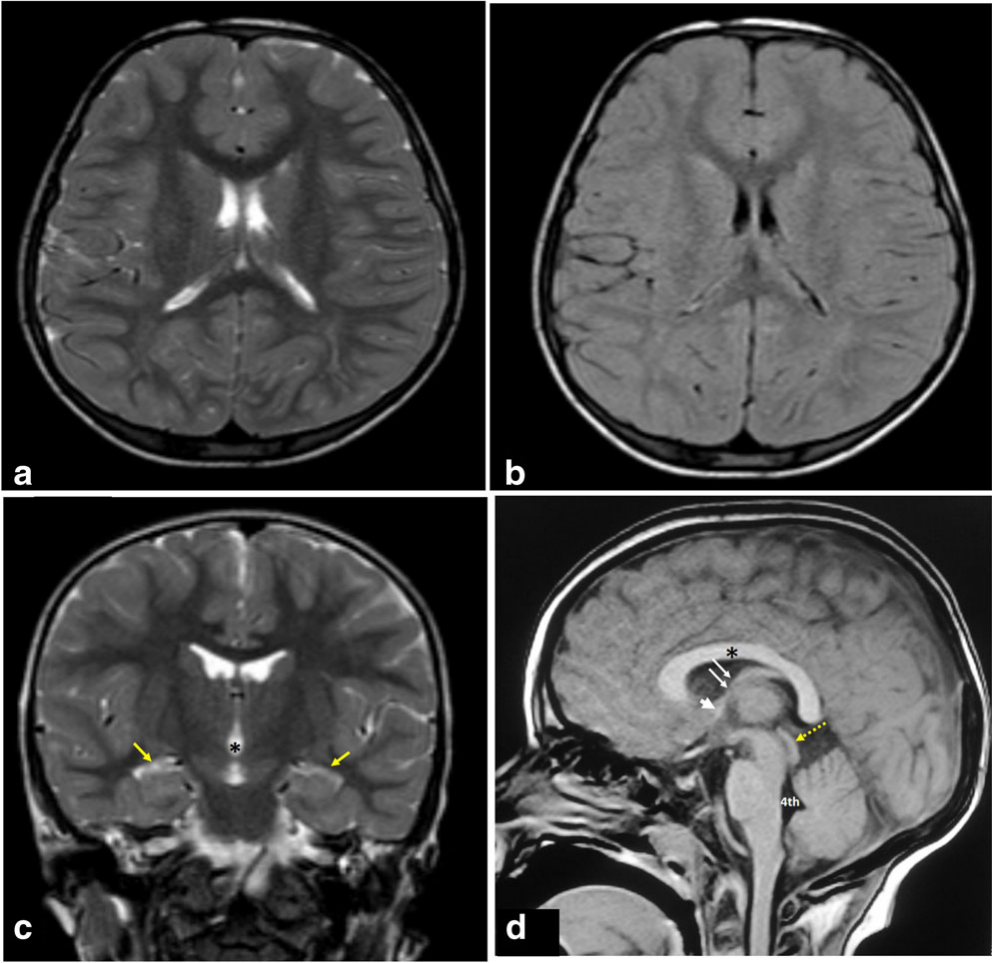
MRI protocol for hydrocephalus. Normal images of a 3-year-old boy: a Axial T2-WI. b Axial FLAIR. c Coronal T2-WI through the lateral and third ventricles (asterisk); note the morphology of the ventricular system, especially the temporal horns (arrows). d Midline sagittal T1-WI image shows the corpus callosum (asterisk), the anterior commissure (short arrow), and the fornix (long arrows). The midbrain with aqueduct and tectal plate (dotted arrow), pons, fourth ventricle (4th), and vermis are well seen
